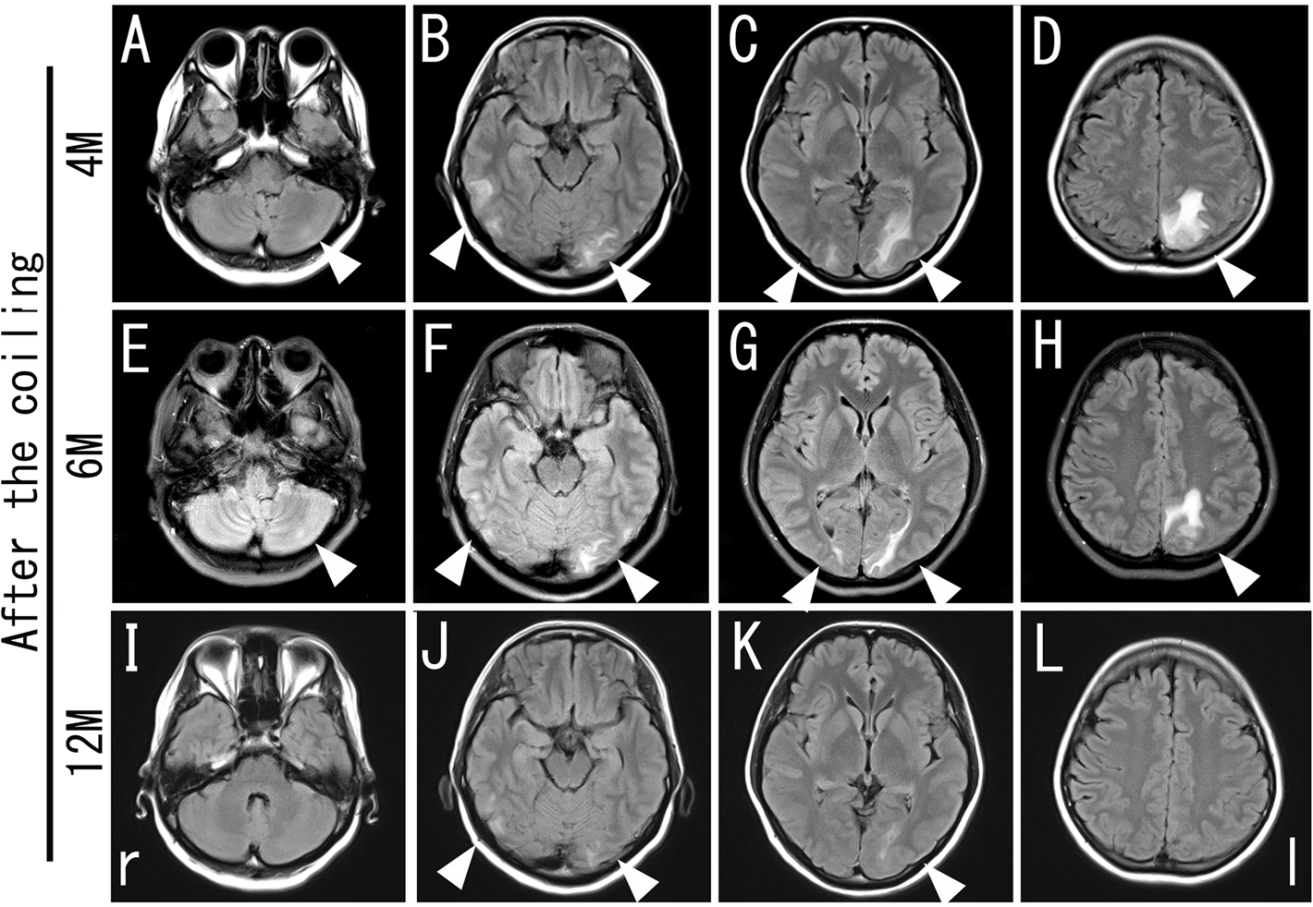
Chronological changes of the brain MRI after the second admission. All lesions observed at 4 months after coiling (A-D, arrowheads) diminished gradually in size at 6 months (E-H, arrowheads) and at 12 months (I-L, arrowheads) after coiling.
